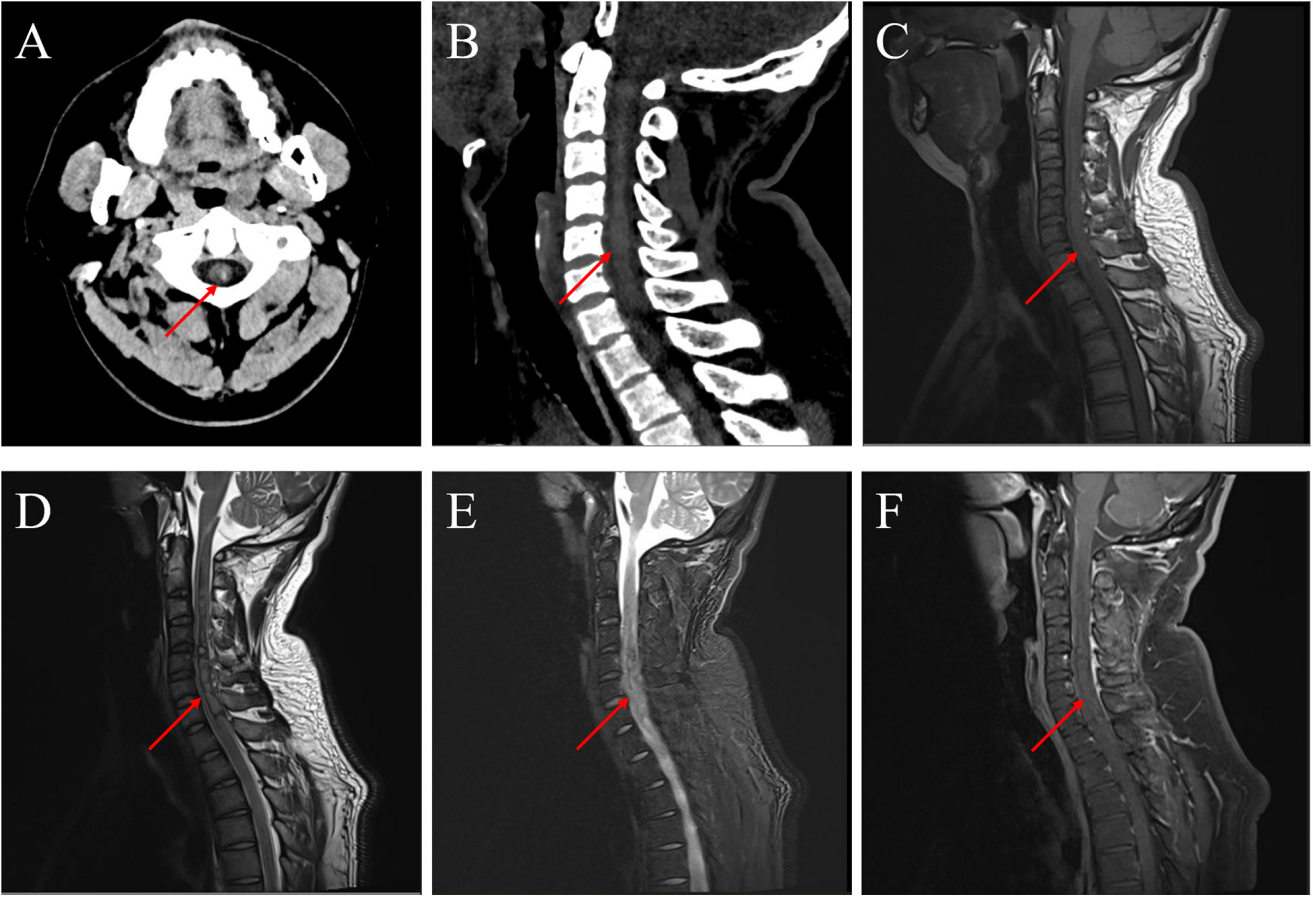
Preoperative imaging. (A) Emergency cranial CT showing medullary hemorrhage. (B) Sagittal view of cervical CT demonstrating the extent of hemorrhage. (C–F) Emergency MRI confirming hemorrhage in the medulla and cervical spinal cord.
